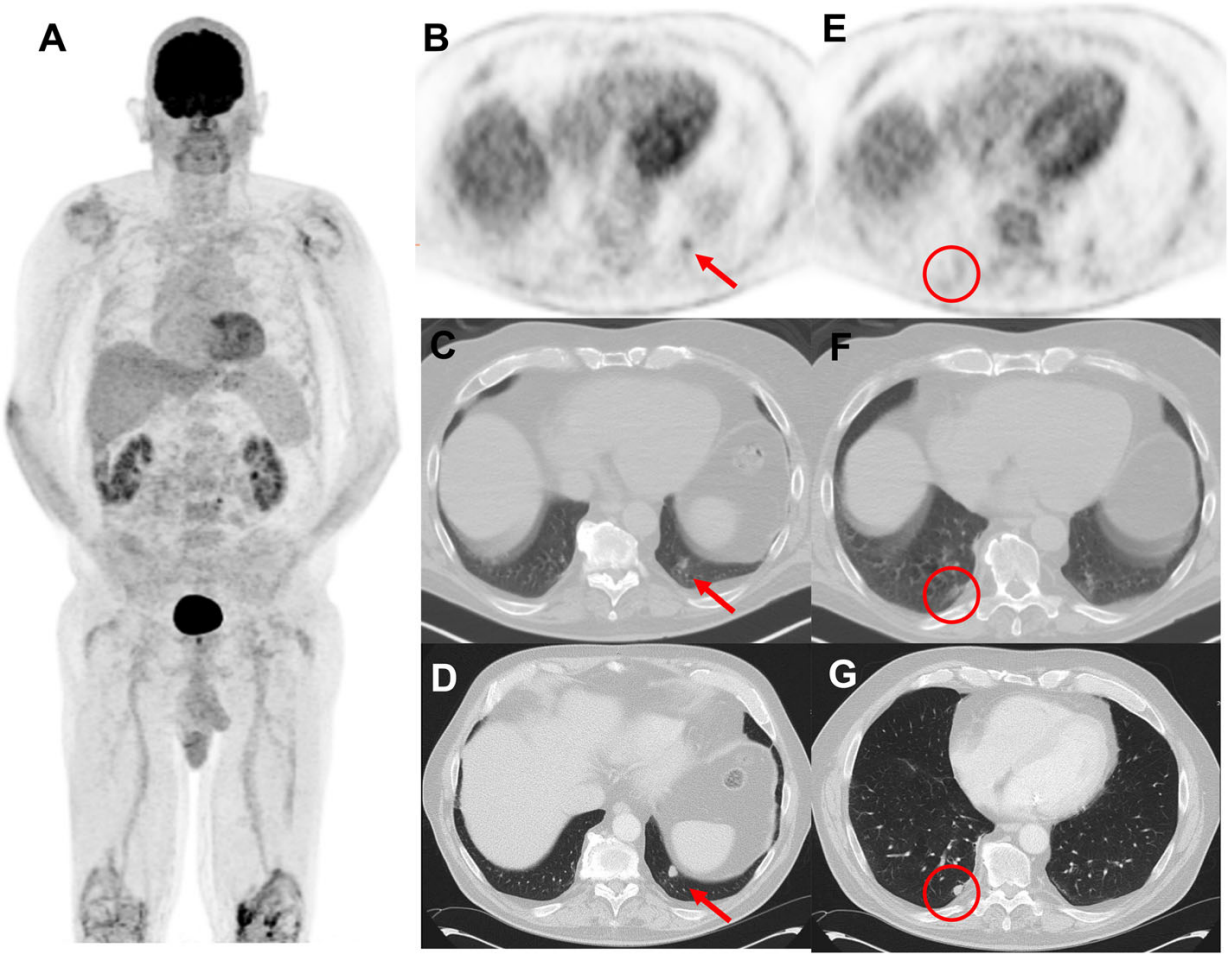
69-year-old man with prior melanoma of the right forearm, 2.1 mm Breslow-depth without ulceration and 1/mm2 mitoses and positive axillary sentinel lymph node (0.2 mm focus with no extracapsular extension) with no adjuvant therapy on observation. Panel A displays surveillance ^18^F-FDG PET MIP 5 months post-resection of the primary lesion. A new FDG-avid nodule was noted in the left lower lobe (panel B and C) which due to respiratory movement appeared ill-defined. A dedicated CT of the chest 7 days later confirmed the nodule in the left lower lobe (panel D) and also showed an additional nodule in the right lower lobe (panel G) which went undetected on the PET/CT (panel E and F) and were suspicious for metastatic melanoma. Wedge resection of the left lower lobe revealed caseation granuloma and the patient continued on surveillance
